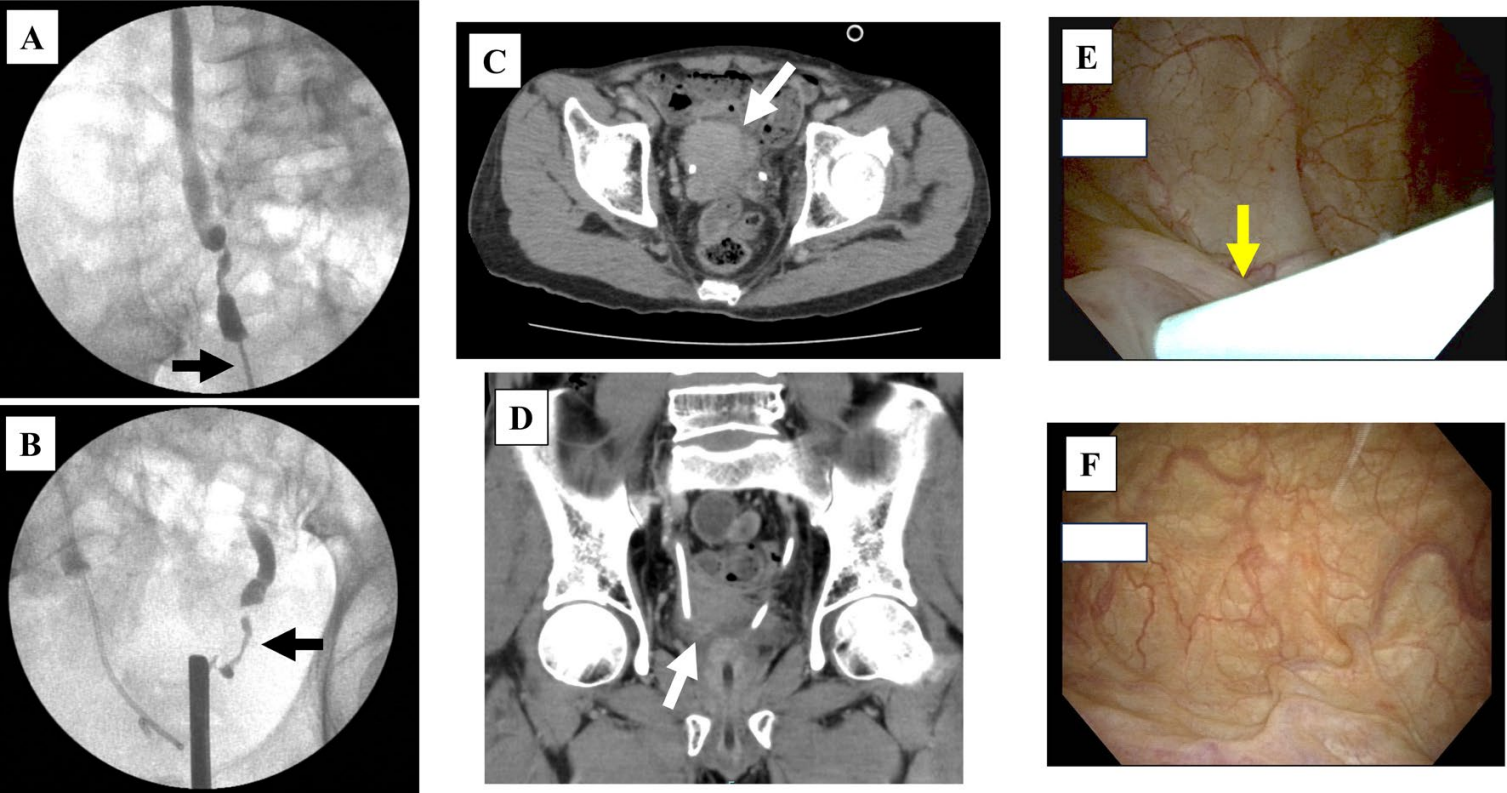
Radiographic and cystoscopic images of the bilateral ureters, submucosal bladder tumor, and bladder mucosa. Retrograde pyeloureterography (A, right ureter; B, left ureter) revealed distal ureteral strictures in both ureters (black arrows). Axial (C) and coronal (D) computed tomography images showed a 50‐mm soft‐tissue mass located at the right dorsal bladder wall (white arrow). (E, F) Cystoscopic examination revealed no mucosal abnormalities at the site of the lesion. The yellow arrow indicates the right ureteral stent.
